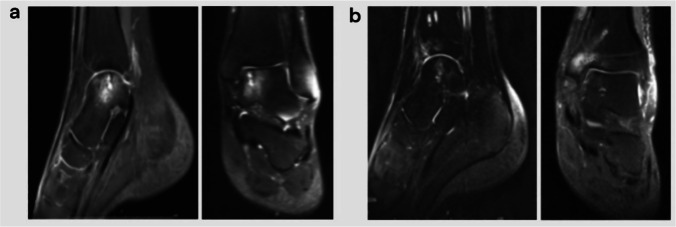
MRI before and at the last follow-up after surgery. a Pre-operative MRI showing cystic degeneration of the medial talus with a local high signal. b MRI of the patient at the last follow-up (17 months after surgery), after talus osteochondral transplantation; there is no obvious change in the medial talus. Cystic degeneration and high signal intensity were significantly reduced
